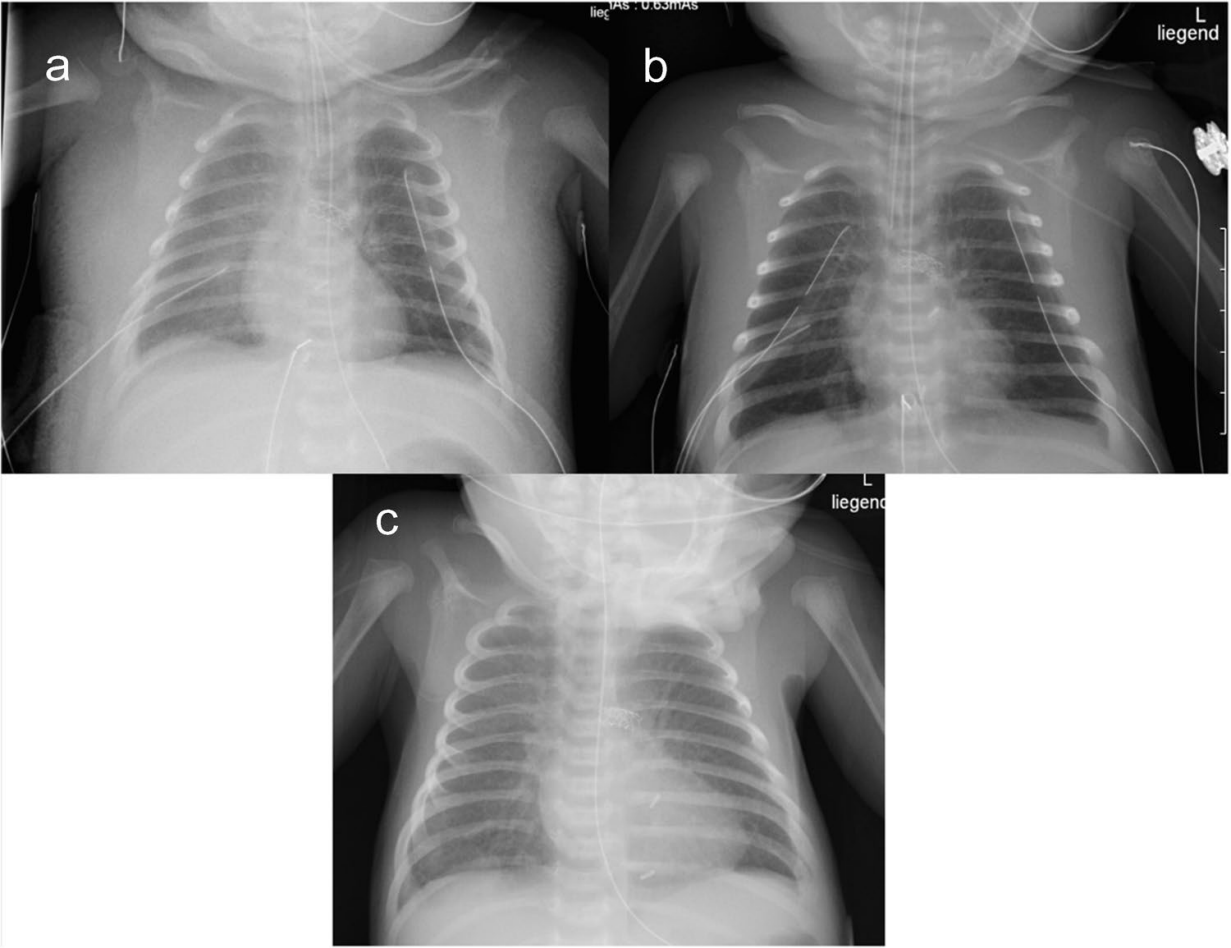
Serial chest radiographs in a 3-kg female neonate with a high-output chylothorax. a Day 16 of life: 1 day after percutaneous catheter intervention. Anteroposterior chest radiograph shows anasarca, pulmonary edema, and a right-sided pleural effusion. The patient is intubated, and thoracic and pericardial drains are in place. b Day 23 of life: 4 days after percutaneous thoracic duct access and mechanical thrombus clearance. Chest radiograph in anteroposterior projection shows resolving anasarca, pulmonary edema, and pleura effusion. Thoracic and pericardial drains remain in place. c Day 31 of life: 12 days post-intervention: Anteroposterior chest radiograph shows further improvement in anasarca. The child is extubated; thoracic and pericardial drains have been removed
